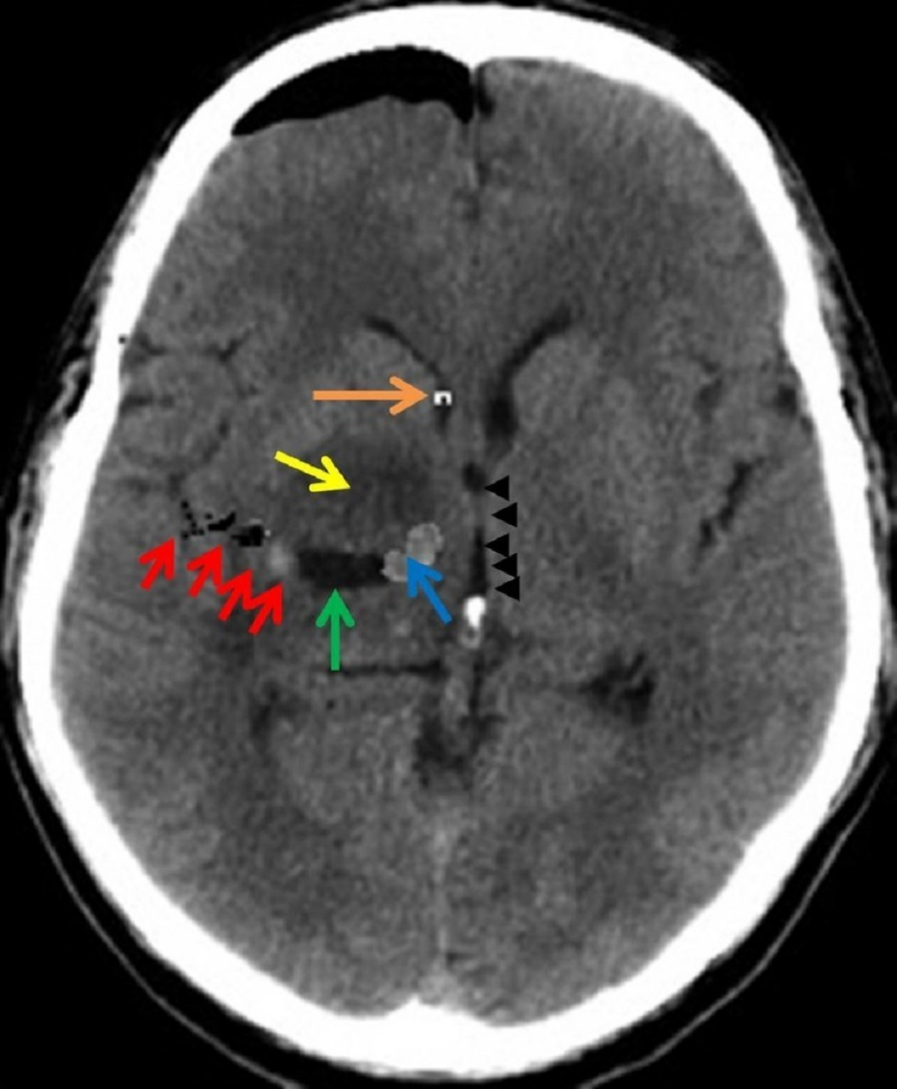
Postoperative Computed Tomography (CT) Scan of Patient Postoperative computed tomography (CT) scan of patient shows the trajectory (red arrows), the resection cavity (green arrow), with some tumor remnant mixed with small hematoma (blue arrow) and edema (yellow arrow). An extraventricular drain (EVD) is visible in lateral ventricle (orange arrow), reducing the hydrocephalus. Note that the midline shift is decreased due to the resection (black arrowheads).
